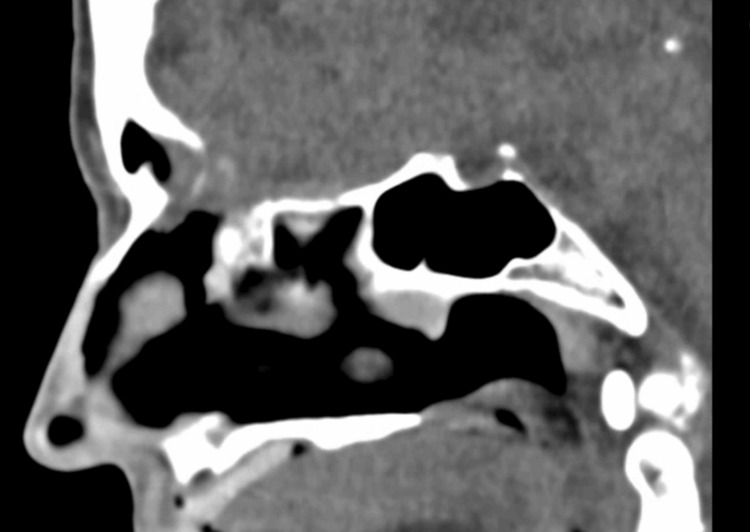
CT of the paranasal sinuses (CT-PNS) showing decreased filling of the left frontal sinus, without clear evidence of significant inflammatory recurrence. A persistent bony discontinuity is noted, with a potential communication to the anterior cranial fossa.
